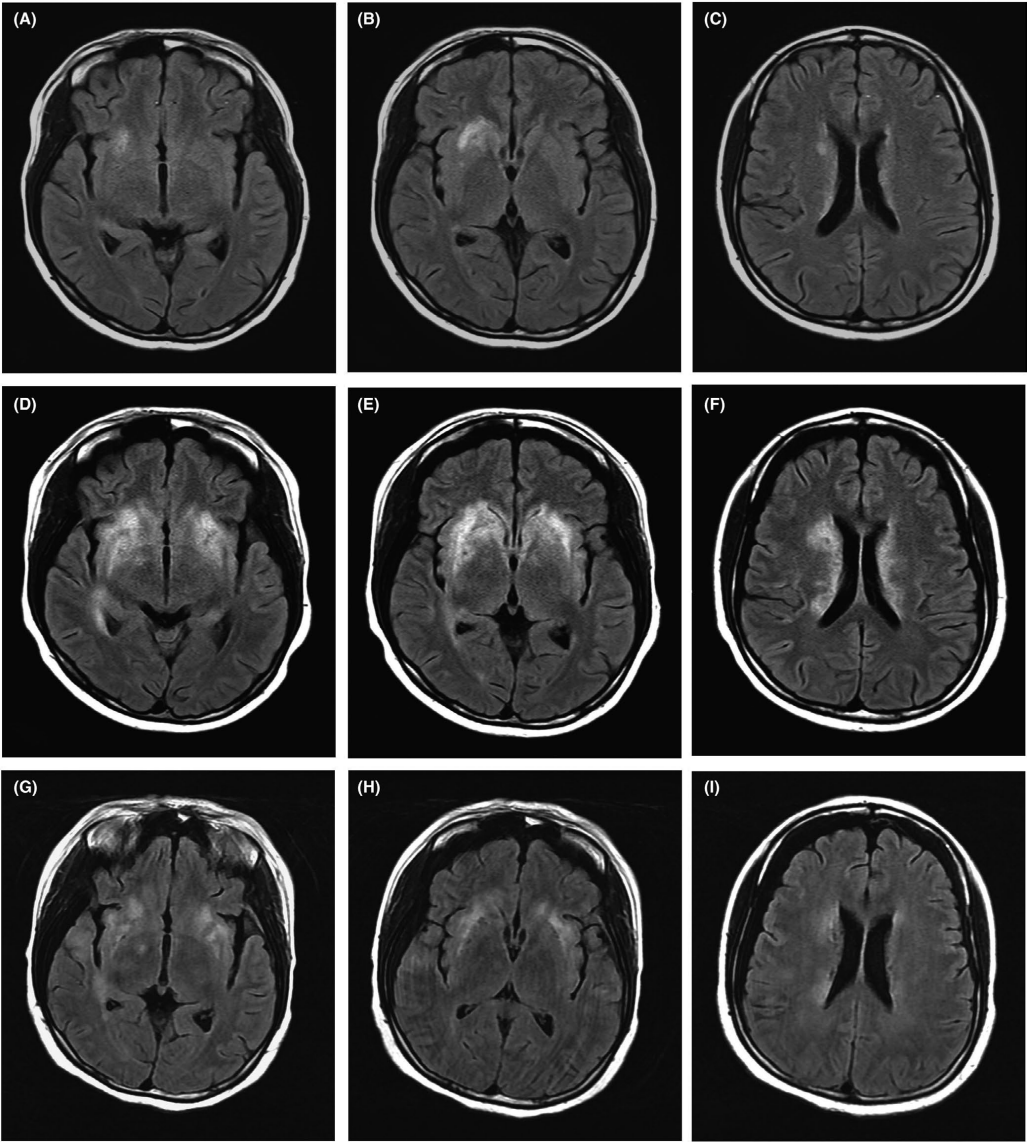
The changes in brain lesions in patient's MR scan during treatment. The abnormal signals could be found in axial fluid‐attenuated inversion recovery (FLAIR) sequences. Patients' first MRI showed diffuse and symmetric hyperintense areas in the bilateral periventricular white matter, basal ganglia, and insula (A‐C). Compared with first MR scan, the area of brain lesions expanded obviously before protein A immunoadsorption treatment (D‐F). After two sessions of protein A immunoadsorptions, FLAIR images of MRI decreased significantly area of lesions (G‐I).
